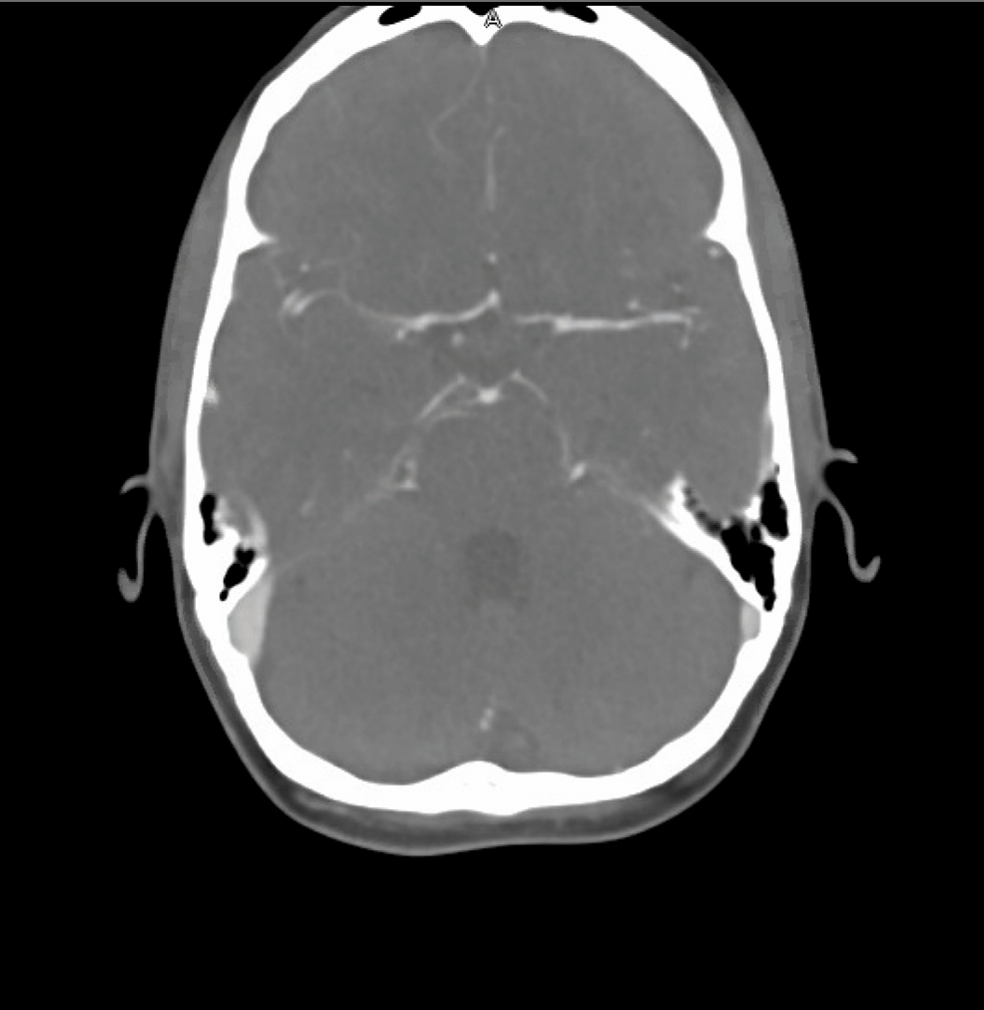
CT angiography of the head and neck showing no evidence of stenosis, large vessel occlusion, or aneurysm.
